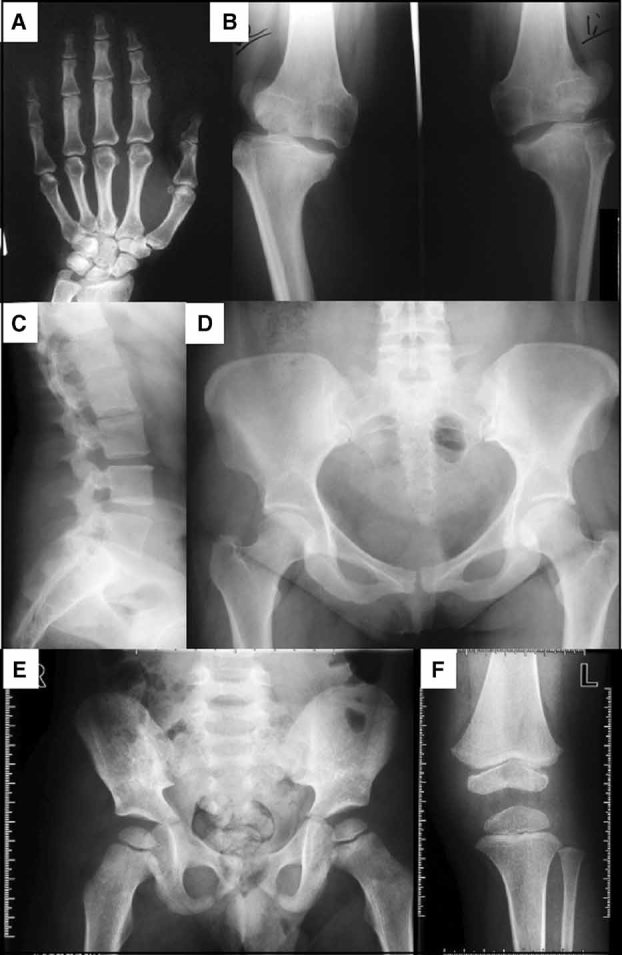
Radiographs of the proband (individual III-4, panels A–D) in Family 1 at 33 years of age and her eldest son (individual IV-6, panels E,F) at 3 years of age. The mother's radiographs showed (A) normal hand, (B) knees with bilateral *genu varus*, irregular joint surfaces, and decreased joint space, especially of the right knee, (C) lateral spine with irregular endplates of the vertebral bodies in the thoracolumbal region and (D) relatively normal hips. Radiographs of her 3-year-old son showing (E) hips and (F) the left knee. The radiographs were consistent with a diagnosis of mild MED, characterized by delayed ossification of the epiphyses. The hips were relatively spared but had small proximal femoral epiphyses, while the knees had small femoral and tibial epiphyses.
